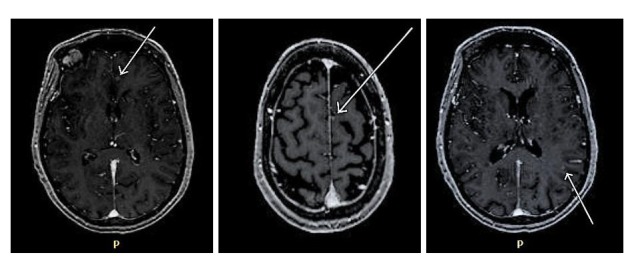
Follow-up MRI of the brain showing new punctate enhancement in the left frontal and parietal region. MRI: Magnetic resonance imaging.
